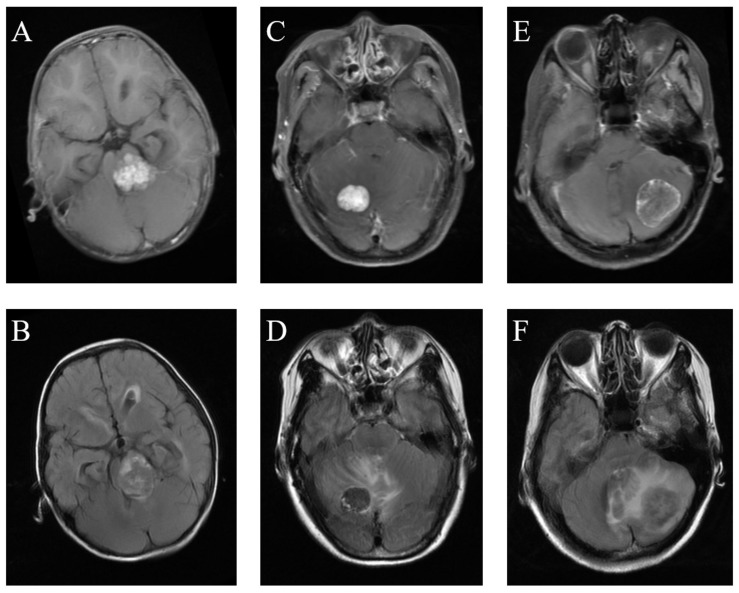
Comparison of MRI among medulloblastoma, hemangioblastoma, and brain metastatic tumor. (A) Medulloblastoma on T1C images. (B) Medulloblastoma on FLAIR images. (C) Hemangioblastoma on T1C images. (D) Hemangioblastoma on FLAIR images. (E) Brain metastatic tumor on T1C images. (F) Brain metastatic tumor on FLAIR images.
